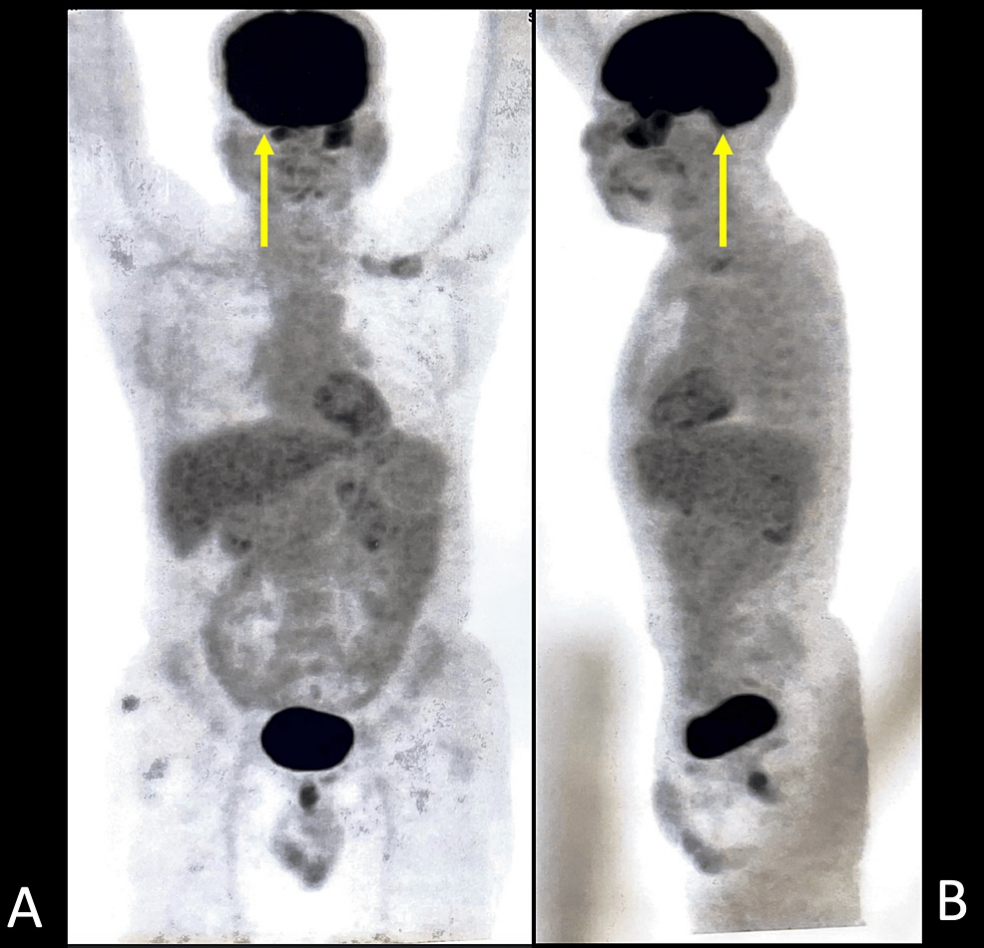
Positron emission tomography-computed tomography scan A. Coronal positron emission tomography-computed tomography scan showing increased metabolic activity in the head region (yellow arrow). B. Sagittal Positron emission tomography-computed tomography scan showing increased metabolic activity in the head region (yellow arrow).
